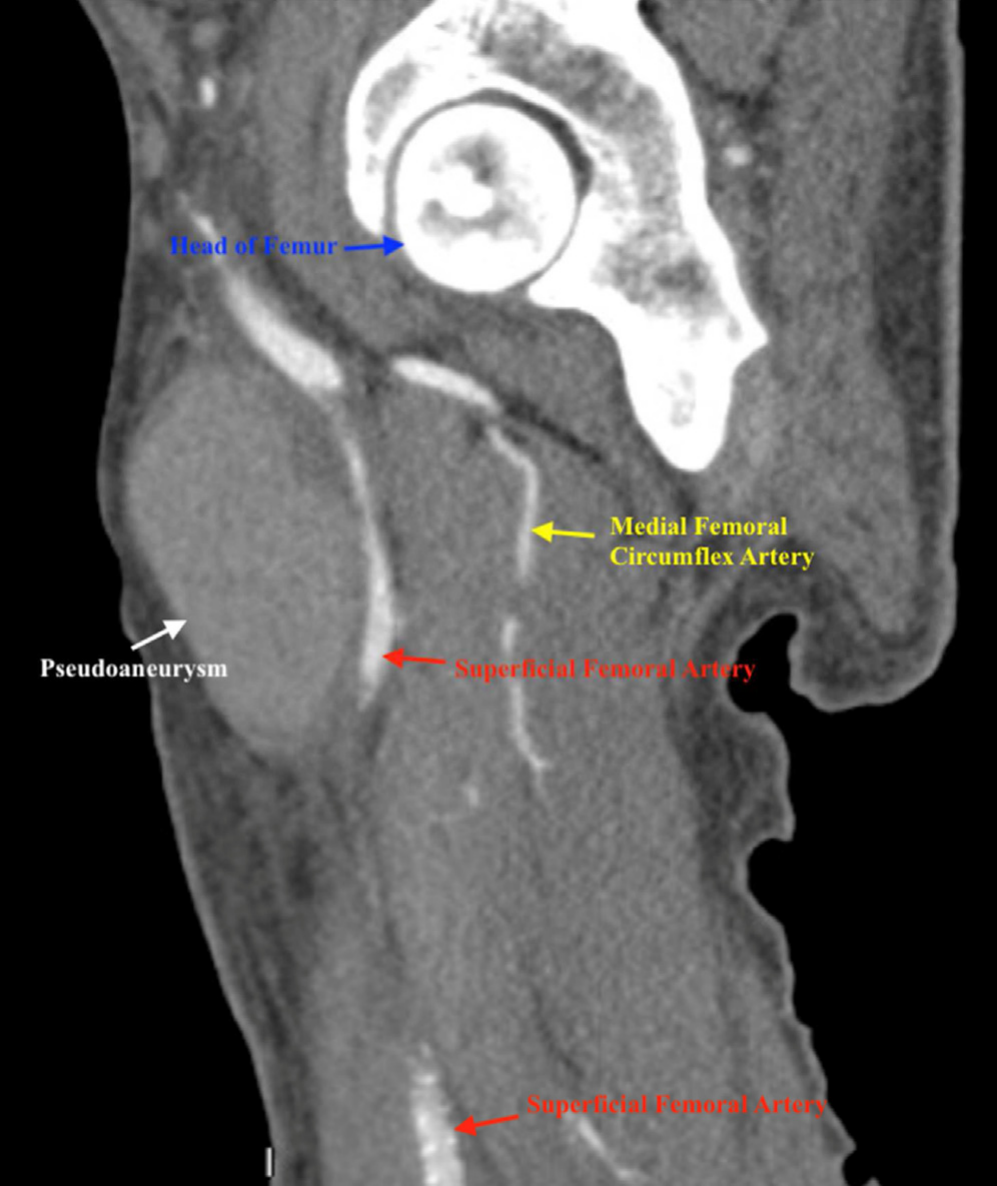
Computed tomography angiogram showing pseudoaneurysm, superficial femoral artery, and medial femoral circumflex artery.
